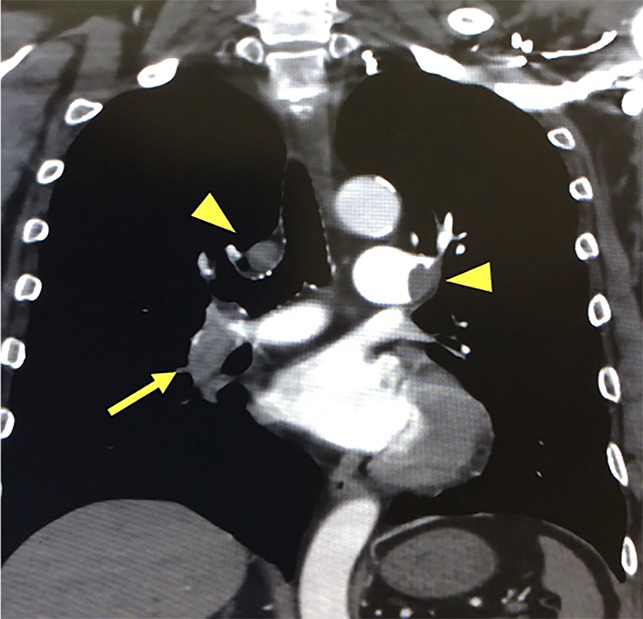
Enhanced CT images demonstrating embolization. Arrowheads: emboli in the bilateral subclavian arteries, arrow: embolus in the right pulmonary artery.
